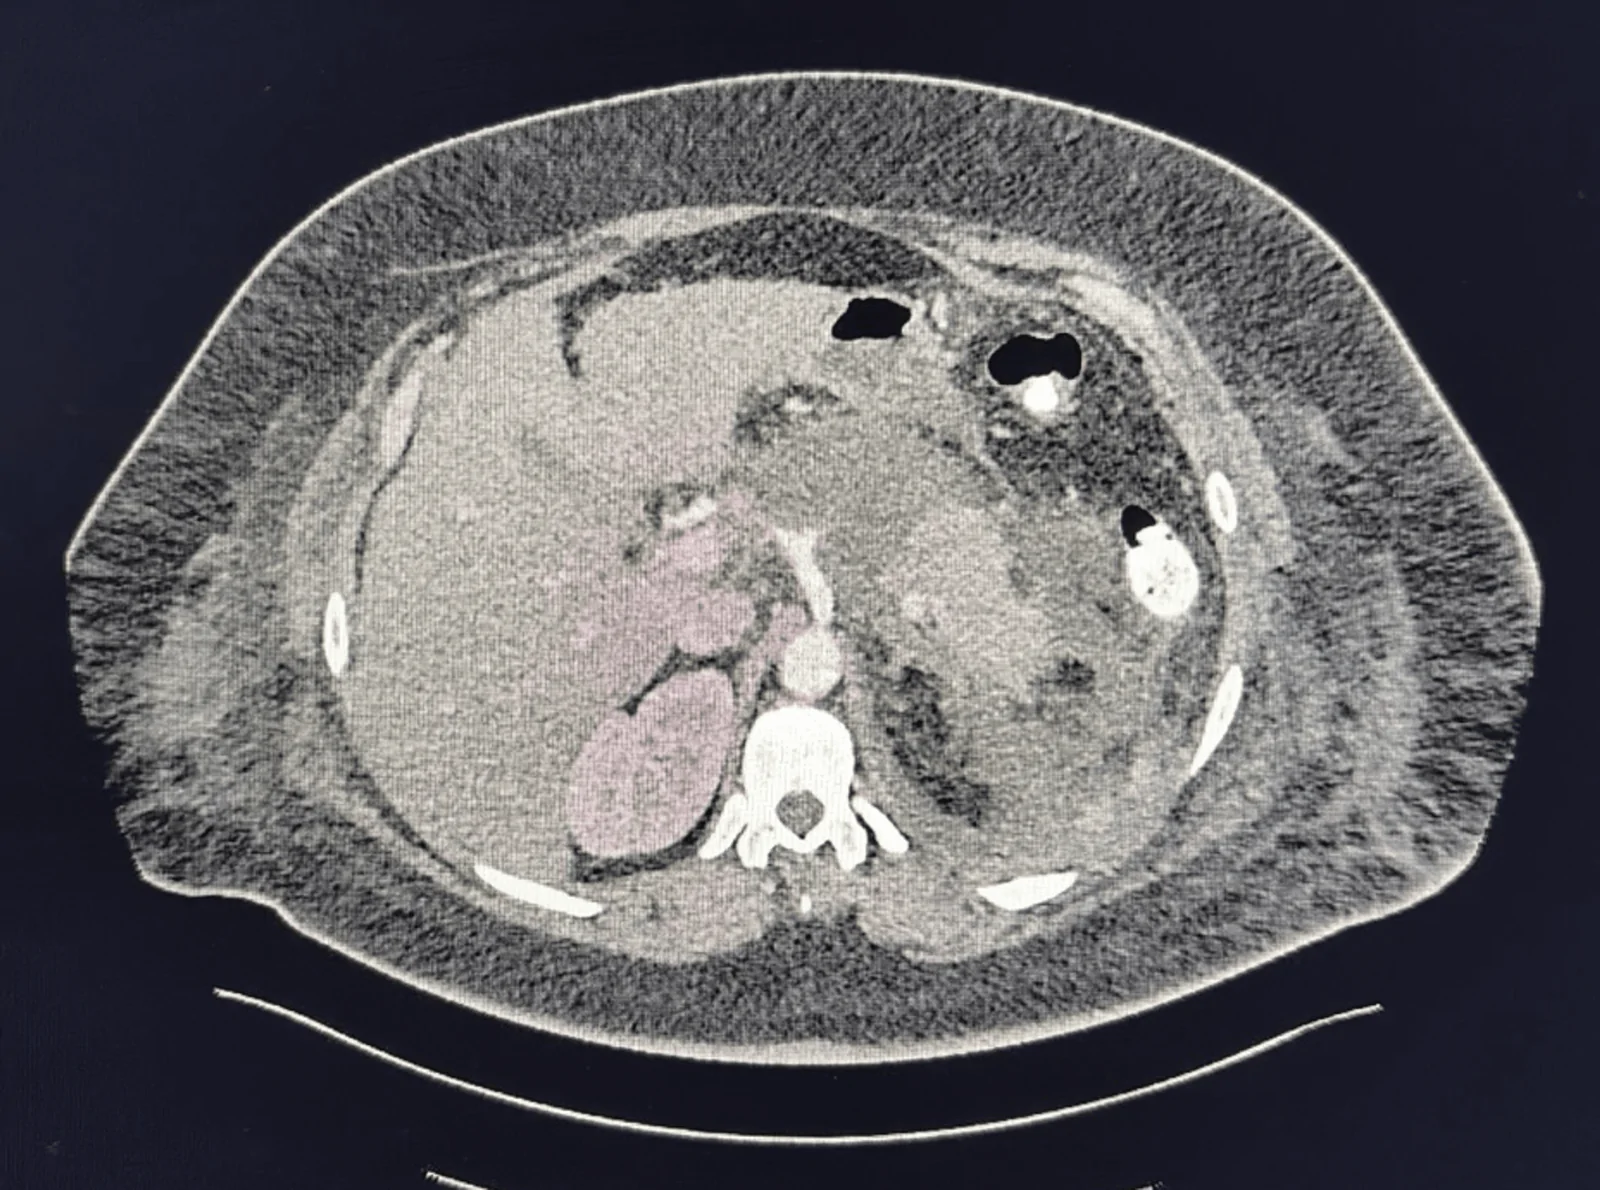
Contrast-enhanced abdominal CT scan, late phase, performed 10 days later after admission. Contrast-enhanced abdominal axial CT scan demonstrating areas of pancreatic necrosis.
